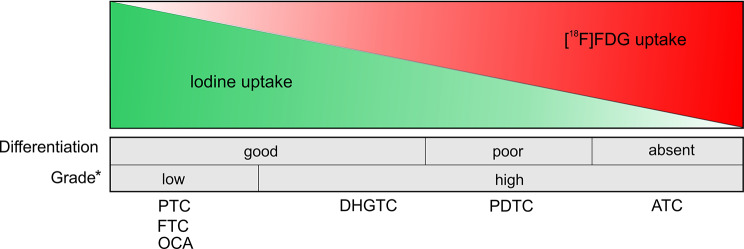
The “flip-flop” phenomenon of radioactive iodine and [^18^F]FDG uptake in follicular cell-derived thyroid carcinoma. Well-differentiated thyroid carcinomas with no high-grade features show high radioactive iodine uptake and low [^18^F]FDG uptake. By contrast, dedifferentiated (anaplastic) thyroid carcinomas are characterized by high [^18^F]FDG uptake and lack of radioactive iodine uptake. Differentiated high-grade thyroid carcinomas and poorly differentiated thyroid carcinomas may exhibit heterogenous uptake of both radiotracers. *mitotic activity, tumour necrosis
